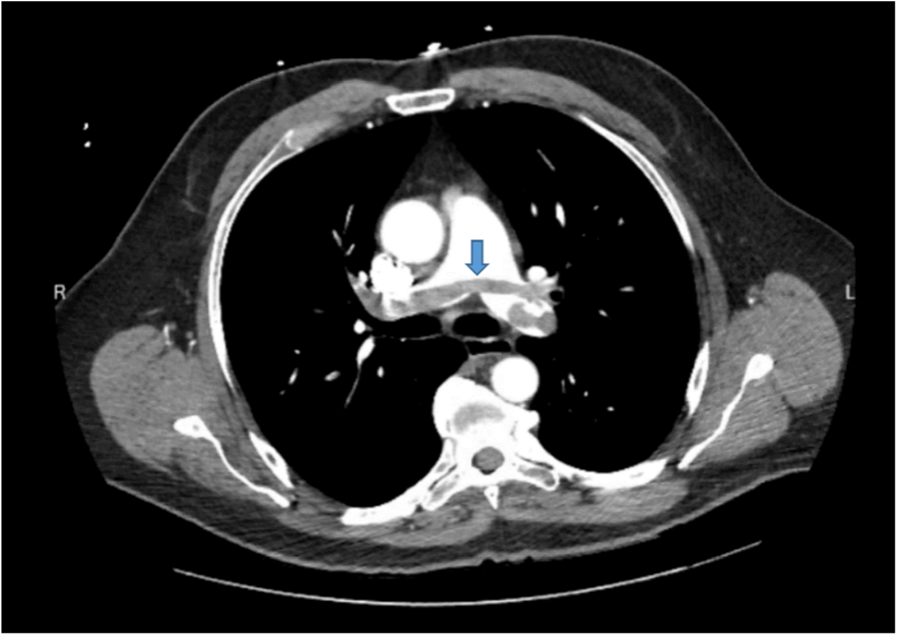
Computed tomography (CT) angiogram of the chest showing large, bilateral pulmonary emboli with a saddle embolus (blue arrow)
